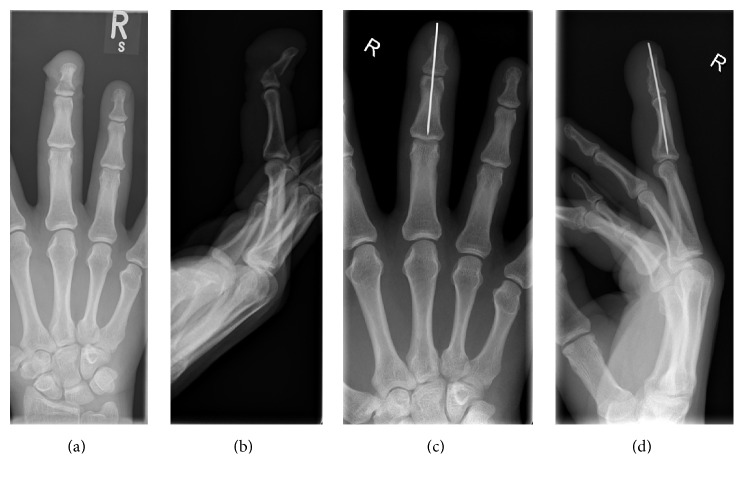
X-rays showing displaced oblique fracture of right middle finger distal phalanx (a, b), which was treated with Kirschner wire fixation (c, d).
